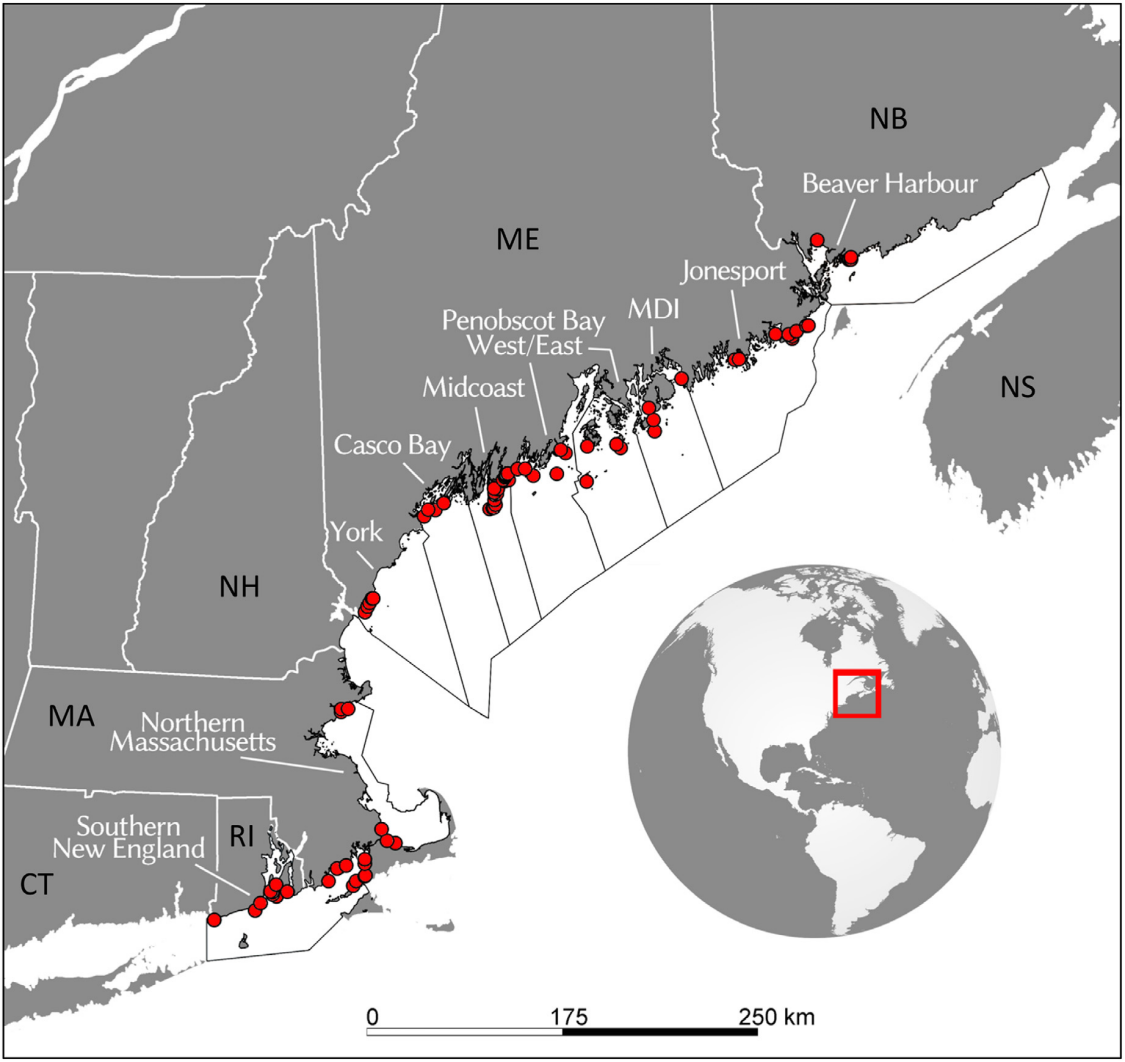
American Lobster Settlement Index sites (red) within study areas (labeled in white); corresponding statistical reporting areas outlined in black. CT, Connecticut, USA; RI, Rhode Island, USA; MA, Massachusetts, USA; NH, New Hampshire, USA; ME, Maine, USE; NB, New Brunswick, Canada; NS, Nova Scotia, Canada.
